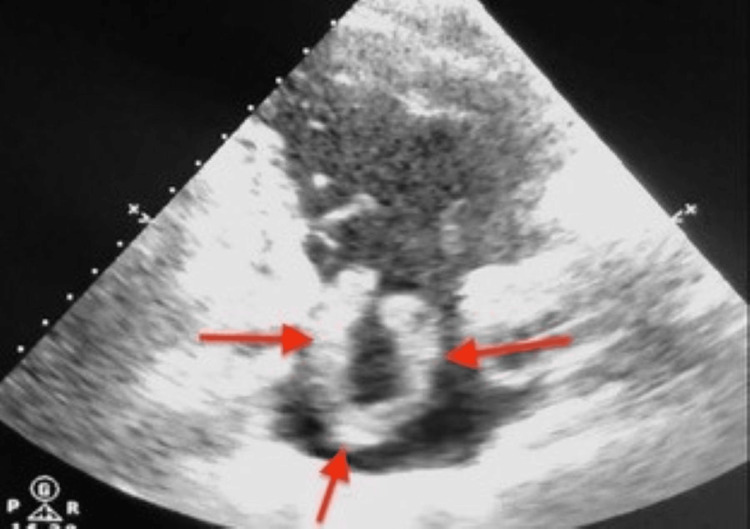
Transthoracic echocardiography, parasternal long-axis view centered on right cavities: visualization of the serpentine thrombus tail within the right atrium (red arrows), confirming its free-floating nature without mural attachment
